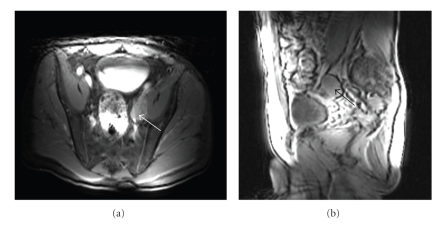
Axial and sagittal magnetic resonance images showing an enlarged left external iliac lymph node (white arrow axial, black arrow sagittal).
